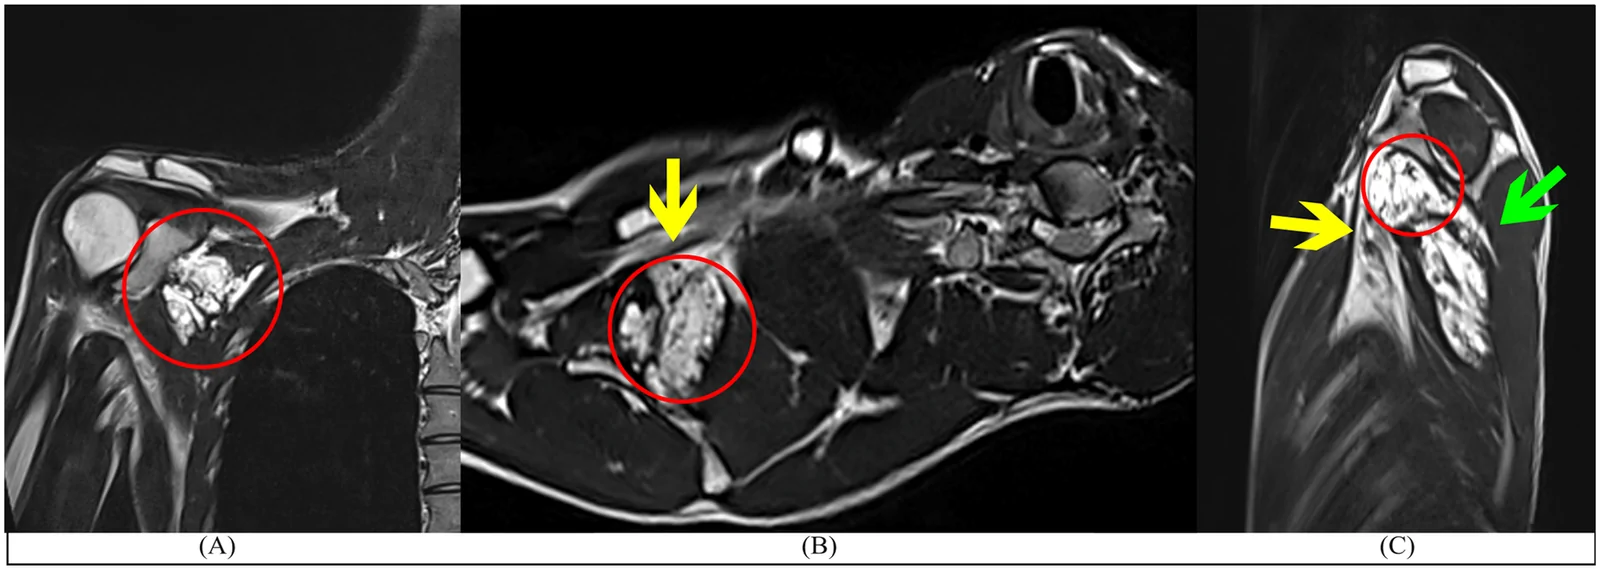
Initial MRI showed a lobulated lesion (red circle) within the right subscapularis muscle measuring 3.7 cm × 6.0 cm × 8.3 cm (AP × W × CC) with hyperintense signal in T2W. The lesion is in close proximity and compressing the cords of the brachial plexus particularly the posterior and lateral cords (yellow arrow). Posteriorly, the lesion is indenting onto the scapula spine (green arrow).
